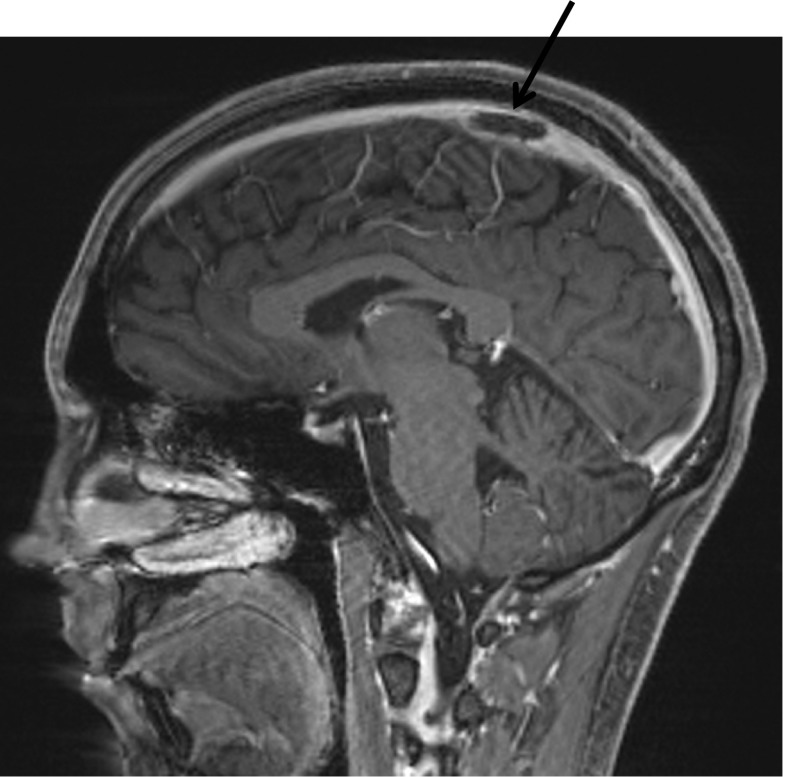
MRI demonstrating superior sagittal sinus thrombosis
